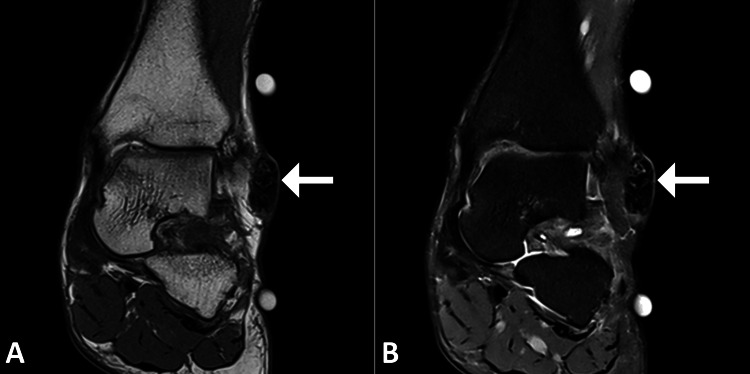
MRI images of the left ankle Coronal images of the ankle in PD-weighted (A) and T2-fat-saturated (B) images show an ovoid lesion over the lateral aspect of the ankle with heterogeneous signal intensity (arrow). MRI: magnetic resonance imaging
